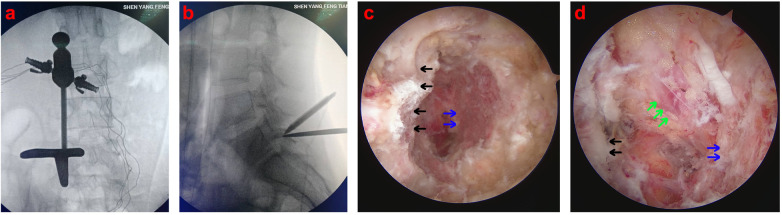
Intraoperative anteroposterior (a) and lateral (b) X-rays confirmed that the channel was located at the isthmus. L5 TP (black arrow) and the sacrum ala (blue arrow) was partially removed (c), and the compression on the left L5 nerve root (green arrow) has been relieved (d).
